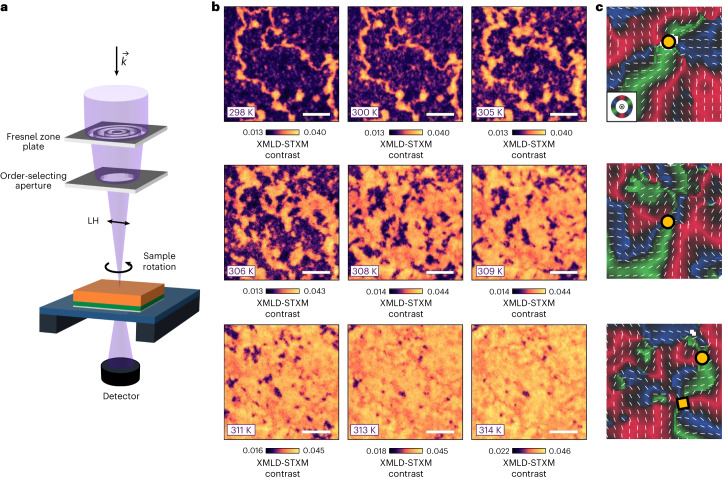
Morin transition and generation of topological AFM textures. a, Geometry of the STXM measurement, performed using linearly polarized X-rays ($$\overrightarrow{k}$$) that are normally incident onto the sample. b, Temperature evolution of the AFM STXM contrast obtained at the Fe L_3_-edge whilst warming the buffered membrane (type C) across *T*_M_, in the temperature range of 298–314 K. The OOP and IP contrasts are indicated in purple and yellow/orange, respectively. Scale bars, 2 µm. All the images were recorded at the same position. The energy-contrast scale was slightly varied across the transition to aid visualization^10^. c, Néel vector maps performed at 314 K (*T* > *T*_M_), produced by rotating the sample azimuth shown in a. The R–G–B colours (key inset in c, top) and thin white bars represent the IP AFM orientations. The white regions represent OOP orientations, whereas the black regions highlight the IP AFM directions, substantially deviating from the R–G–B directions. The yellow circles and squares indicate AFM merons and antimerons, respectively. The width of the images is ∼1.5 μm.
